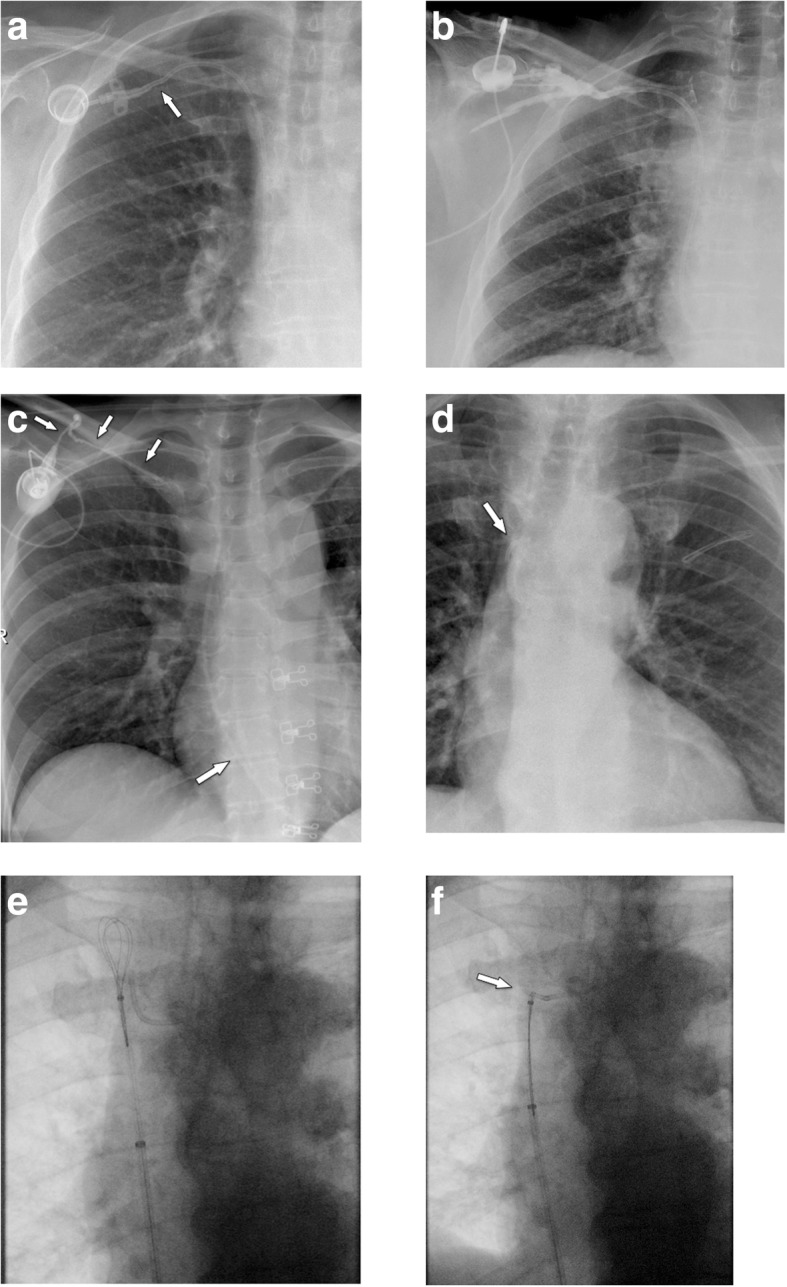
Catheter pinch-off, disconnection, and fracture. a, b Catheter pinch-off with subsequent fracture. a Chest radiographs show a slight kink and pinch-off of the catheter at the thoracic inlet (between clavicle and first rib). b Five months later, a flow confirmation study shows extravasation at the thoracic inlet (due to fracture). Incidental note is made of the catheter tip in the right atrium. c Catheter disconnection in another patient: the entire catheter has gone off and embolized in the heart (large arrow). Only the subcutaneous fibrous tunnel is opacified with contrast (small arrows). d–f Fracture of catheter and percutaneous removal. d After explantation of a port system, a catheter fragment is still visible (arrow). e Via a right femoral vein access a snare is advanced toward the catheter tip. f After pulling the snare tight around the catheter (arrow), it can be safely removed
